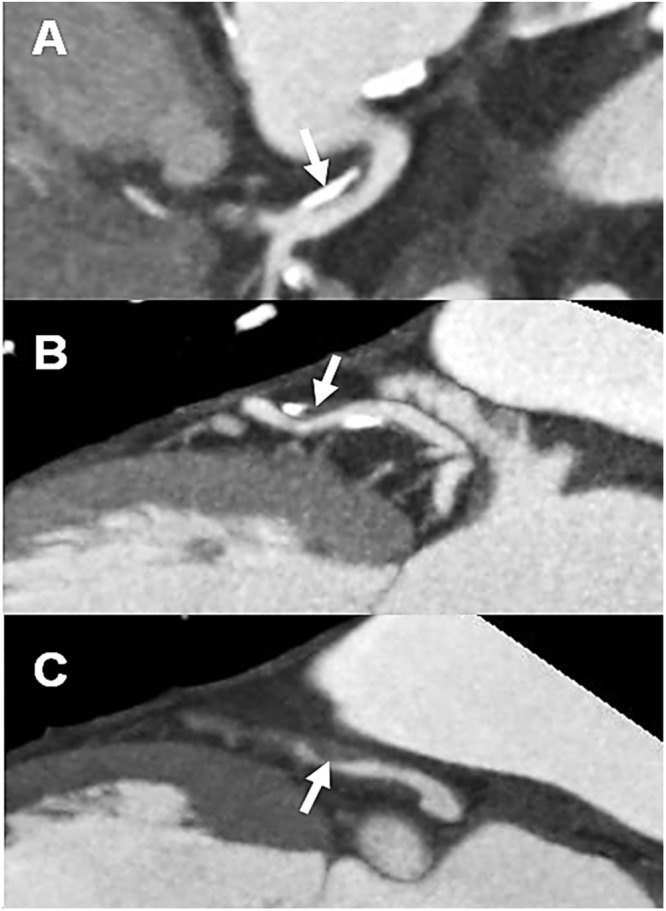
Plaque characteristics of coronary artery on CCTA. Multiplanar reconstruction images demonstrate calcified (A), mixed (B), and noncalcified (C) plaques (arrows) in the proximal left anterior descending coronary arteries. CCTA, coronary computed tomography angiography.
